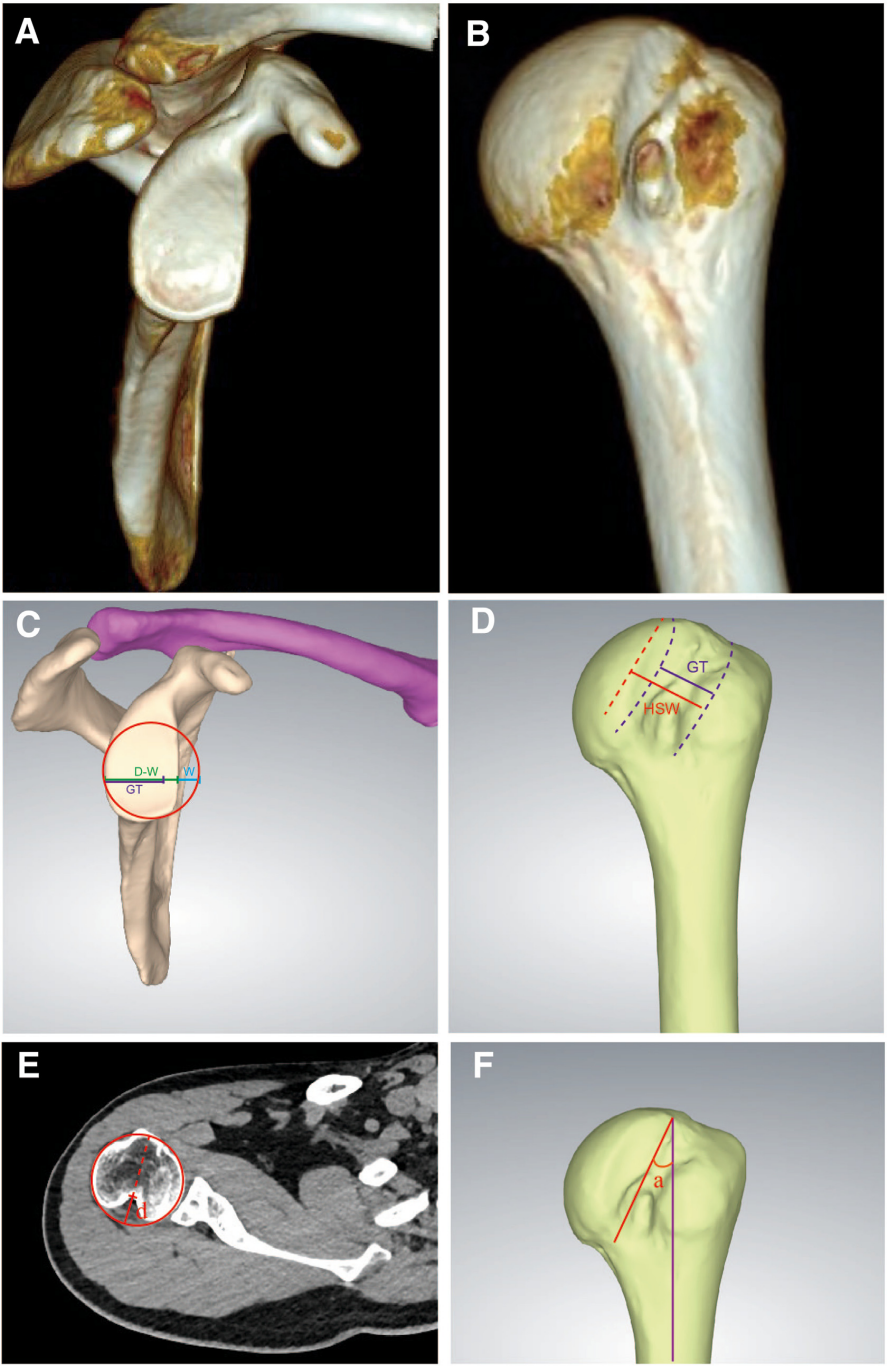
Preoperative computed tomography (CT) scans and 3-dimensional (3D) reconstruction. Shown is the en-face view of right shoulder joint, the glenoid (A), and the posterior view of the humeral head (B) on 3D CT. Computerized 3D construction for the glenoid (C) and humeral head (D). “D” is the diameter of the best-fit circle and the defect width (W) are measured, and “D-W” represents the width of glenoid. The glenoid defect is approximately 23.7%, and therefore the Hill-Sachs bony lesion is defined as “off-track.” The glenoid track (GT) is calculated as 0.83 D-W. “HSW” indicates the width of the Hill-Sachs lesion. (E) On the axial CT image of the humeral head, the diameter of the best-fit circle of the articular surface is measured. In addition, the Hill-Sachs lesion is quantified at its maximal dimension (d). The depth percentage of the Hill-Sachs lesion is found to be 30.9%. (F) The Hill-Sachs angle (a) is defined as the angle formed between the axis of the deepest sulcus of the lesion and the longitudinal axis of the humeral shift.
